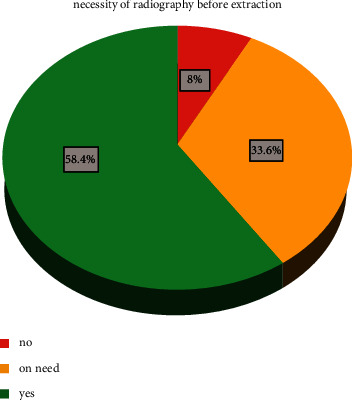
Necessity of radiography before extraction.
